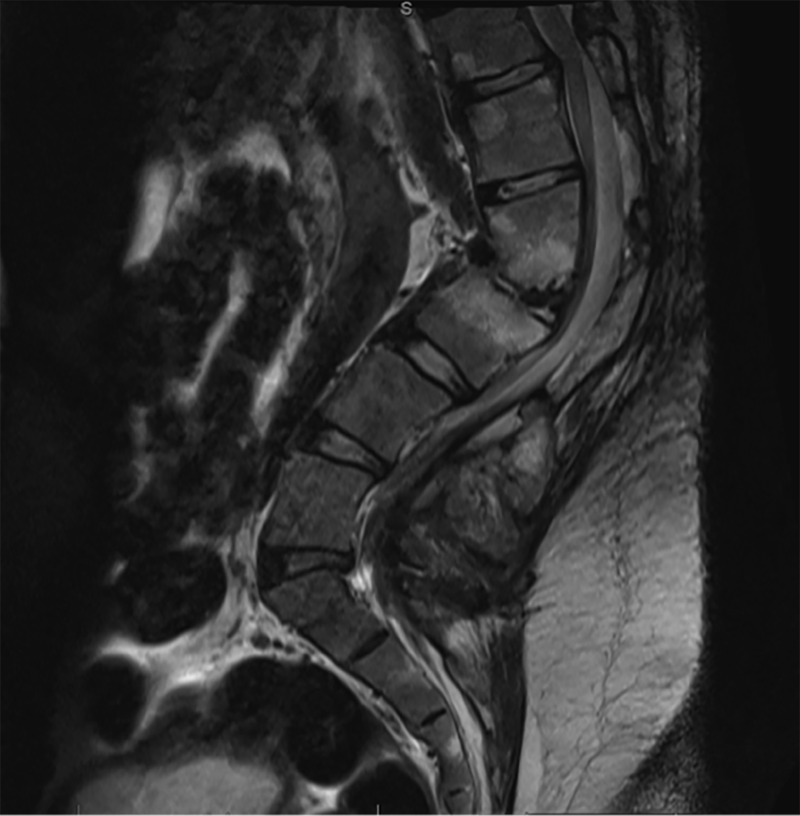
Preoperative Sagittal MRI T2 weighted sagittal MR image demonstrating kyphosis at L2-L3 and spinal stenosis at the area of the pseudoarthrosis
